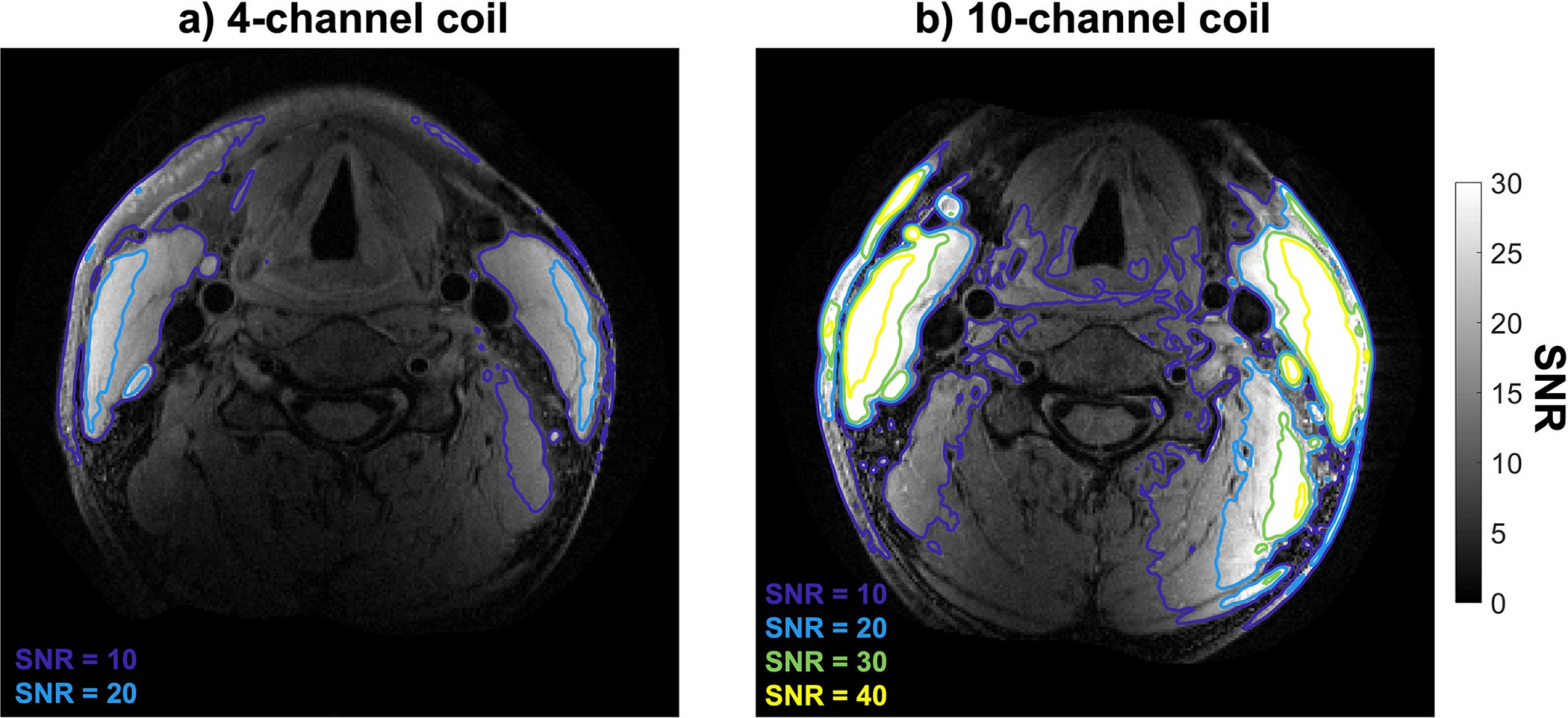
SNR-units reconstructions from transverse T1-weighted turbo-spin-echo data for one healthy volunteer SNR, acquired without parallel imaging acceleration using (a) the 4-channel coil and (b) the 10-channel coil. SNR contours are shown for both acquisitions at iso-contour levels of 10, 20, 30, and 40.
